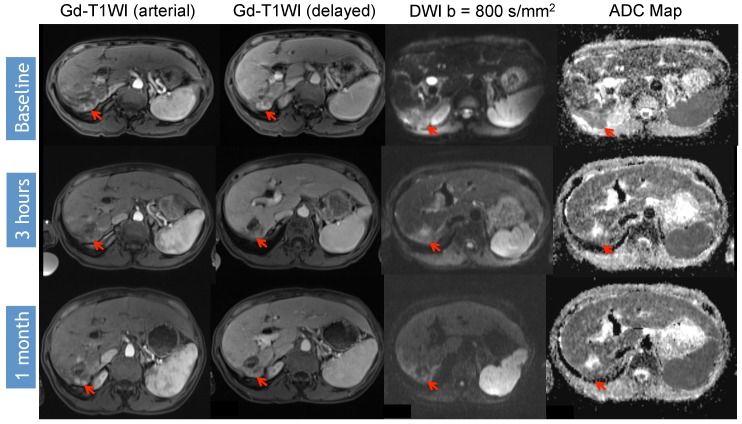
DWI Response Assessment after DEB-TACE treatment. 55-year-old woman presented with a segment VII HCC (arrow) previously treated with DEB-TACE without macrovascular invasion or extrahepatic disease. Note that there is initial absence of enhancement at 3 h, but the area continues to demonstrate restricted diffusion (ADC 0.904 × 10^−3^ mm^2^/s). At one month, the same area now demonstrates enhancement with increased restricted diffusion, findings consistent with progression of disease (PD) (baseline ADC 0.904 × 10^−3^ mm^2^/s, post-3 h ADC 1.02 × 10^−3^ mm^2^/s, post-one month ADC 0.830 × 10^−3^ mm^2^/s).
